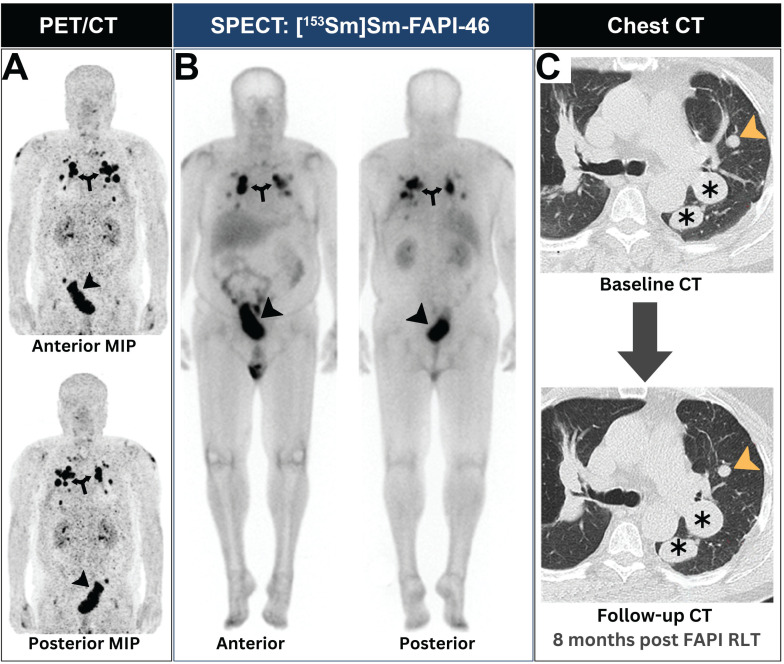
A patient with lung sarcoma achieved successful neoplastic disease control after receiving FAPI RLT. (A) Baseline PET revealed intense [^68^Ga]Ga-FAPI expression at both pulmonary (arrows) and pelvic lesions (arrowheads). (B) SPECT imaging showed adequate scintigraphic localization of [^153^Sm]Sm-FAPI-46 within all neoplastic lesions (annotations). (C) After two [^153^Sm]Sm-FAPI-46 cycles and a single [^90^Y]Y-FAPI-46 cycle, the patient achieved stable disease control in all lesions as evident by chest CTs (annotations). Images were adapted with permission from reference 45, copyright: © 2021 Springer Nature. Published under creative commons (License: CC BY 4.0).
